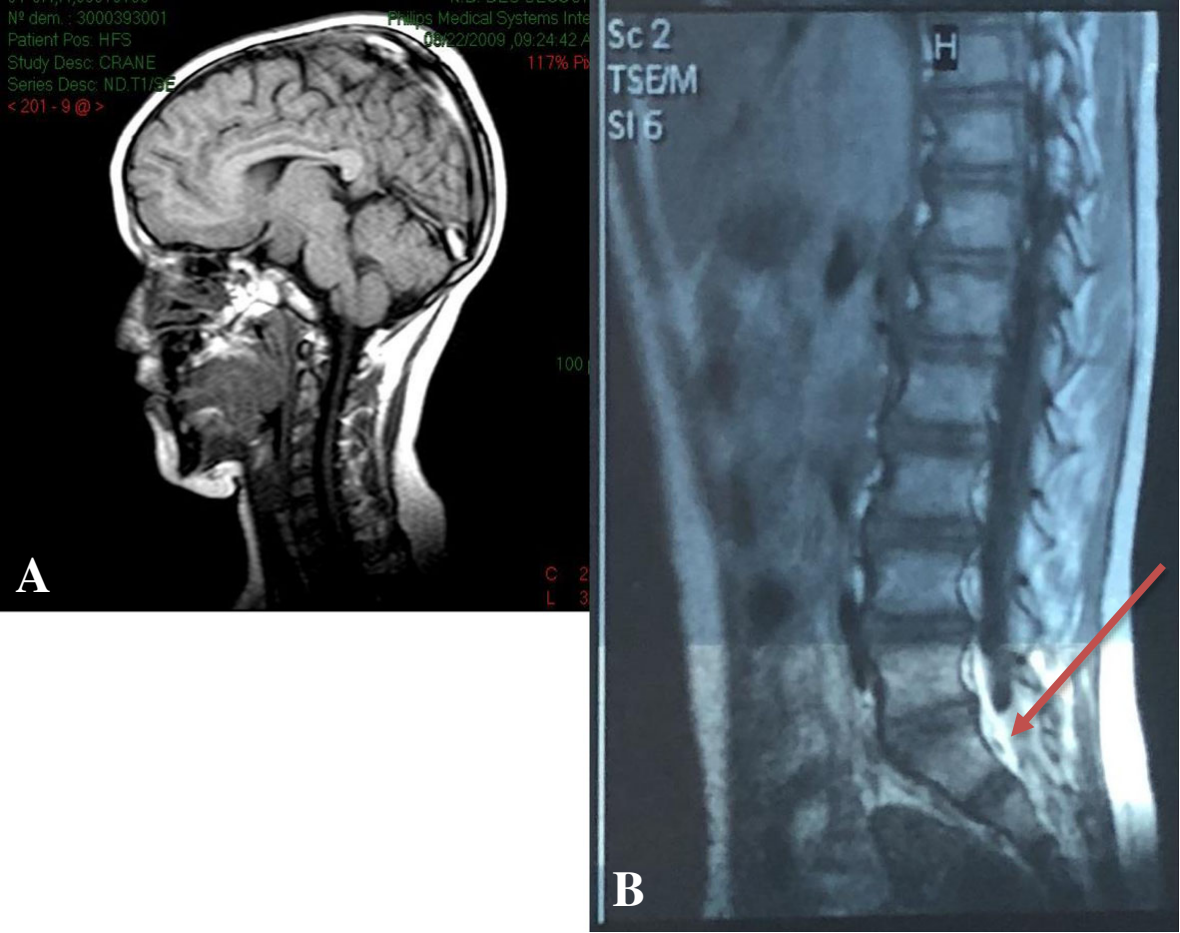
Magnetic resonance imaging of the brain and the spine. a Magnetic resonance imaging of the brain – hypothalamic hamartoma. b Magnetic resonance imaging of the spine (T2-weighted scan) – fibrolipoma of the filum (*red arrow*)
